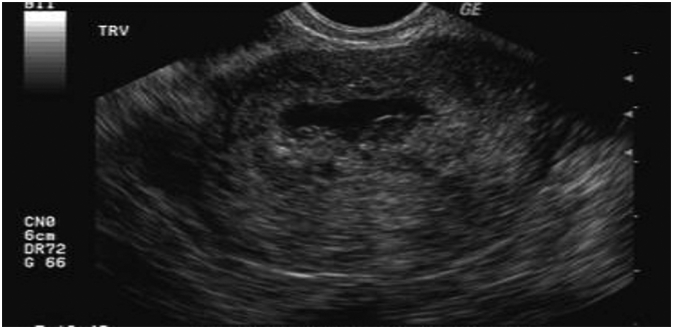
Below ultrasound showing significant retained product of conception in the uterine cavity.
